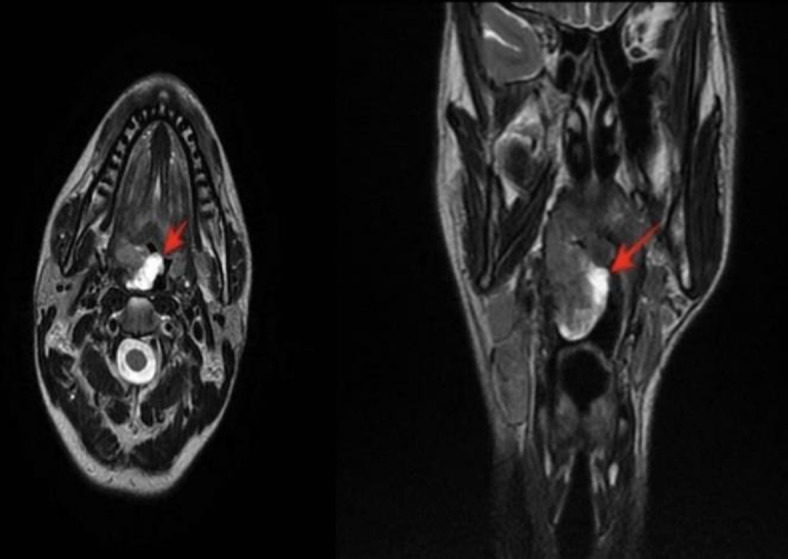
Head and neck contrasted MRI
